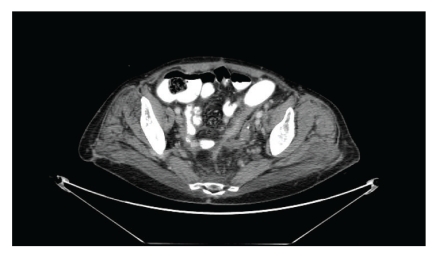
CT scan showing fibrosis of sigmoid.
